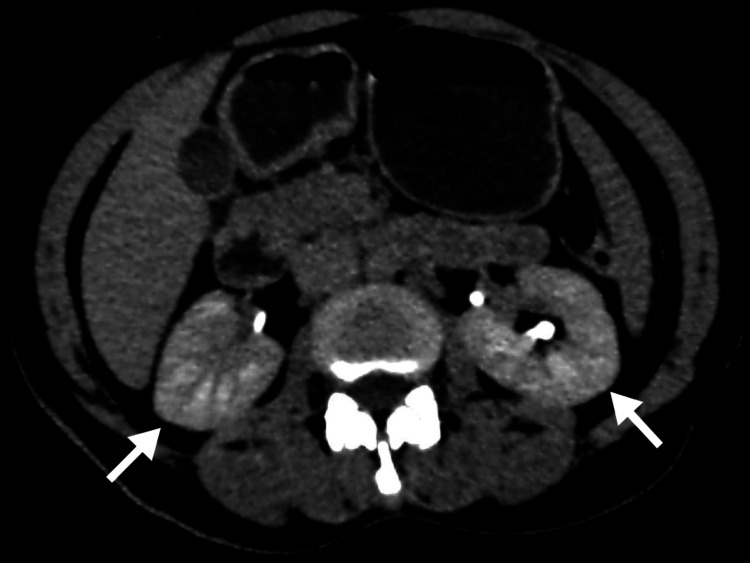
Acute pyelonephritis with striated nephrogram on contrast-enhanced CT. A 55-year-old woman with complaints of right loin pain was diagnosed with acute pyelonephritis. Contrast-enhanced axial CT of the abdomen image of the patient shows a striated nephrogram (arrows). These images are from the hospital and have not been published elsewhere. CT: computed tomography
